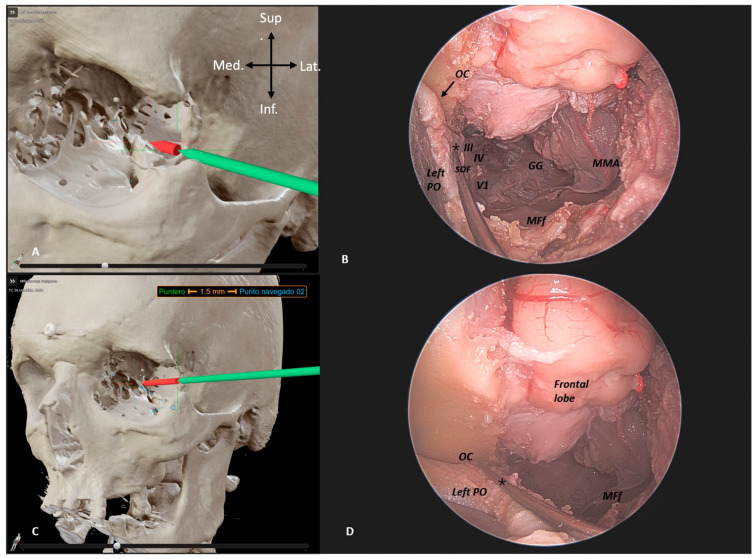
BrainLab^®^ CT reconstruction post left ETOA dissection. (A–C) Neuronavigation-guided distance measurement to key targets in spheno-orbital meningiomas. (B) Superior orbital fissure (SOF, *) with cranial nerves III, IV, V1; Foramen Rotondum (FR) with V2; Gasserian gangion (GG), middle meningeal artery (MMA), and middle fossa floor (MFf). (D) PO, optic chiasm (OC, *), frontal lobe, and MFf. * the pointer identifies the anatomical structure seen in the BrainLab^®^ CT reconstruction.
